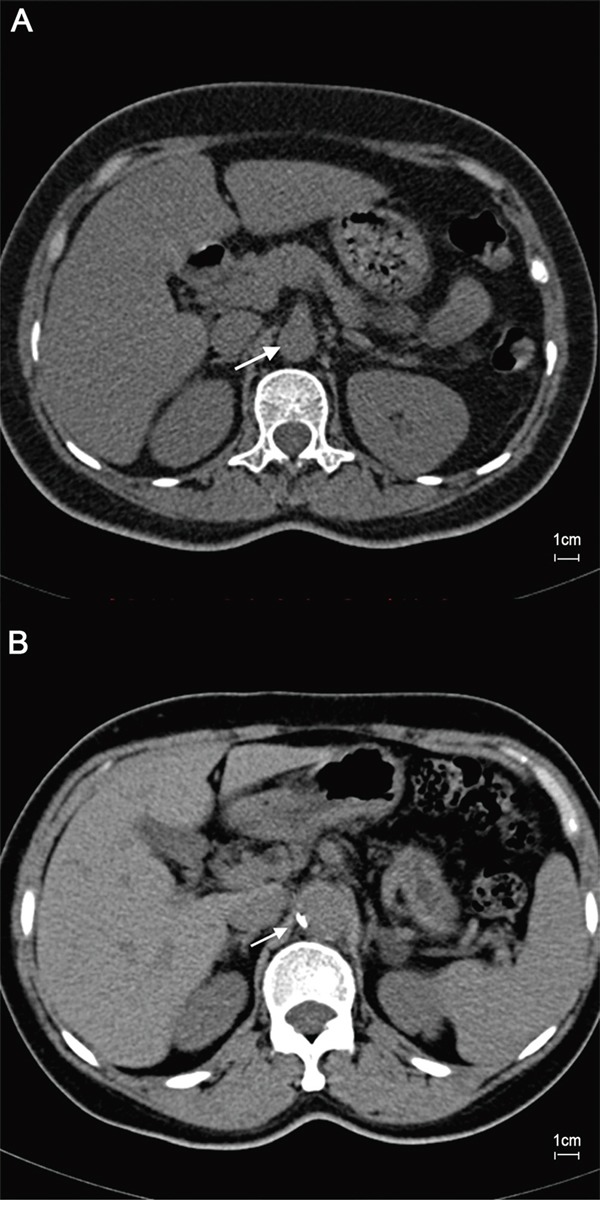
Axial cross-sections were obtained by computed tomography in patients with normal and high ABI The image in a 47-year-old patient with normal ABI showed no obvious calcification (arrow) in abdominal aorta A. However, abdominal aorta calcification was evident (arrow) in a 46-year-old patient with high ABI B. Scale bar=1cm.
